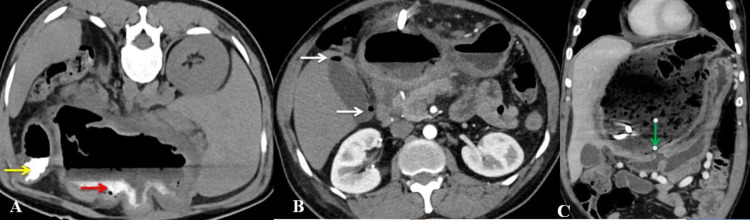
CT of the abdomen A (axial view, prone position): Oral contrast into the stomach (yellow arrow) and the cyst (red arrow)
